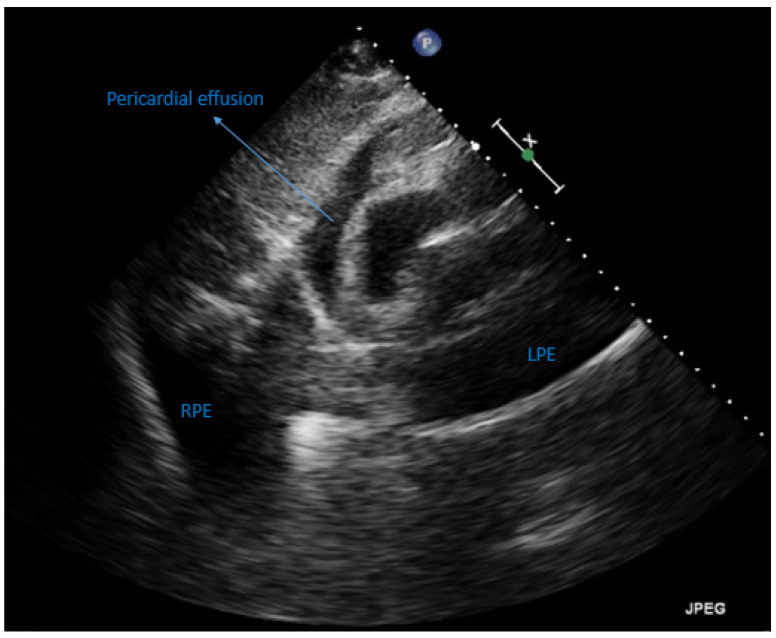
Transthoracic echocardiography captured on the 45th day during hospitalization. The subcostal view shows a small amount of pericardial effusion and massive refractory pleural effusion bilaterally. LPE = left pleural effusion; RPE = right pleural effusion.
